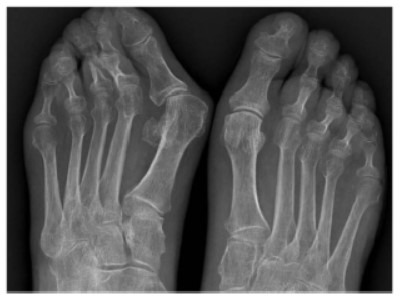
Initial radiograph of the feet, showing no abnormalities in the fifth toe of the right foot. Taken on January, 2024.
